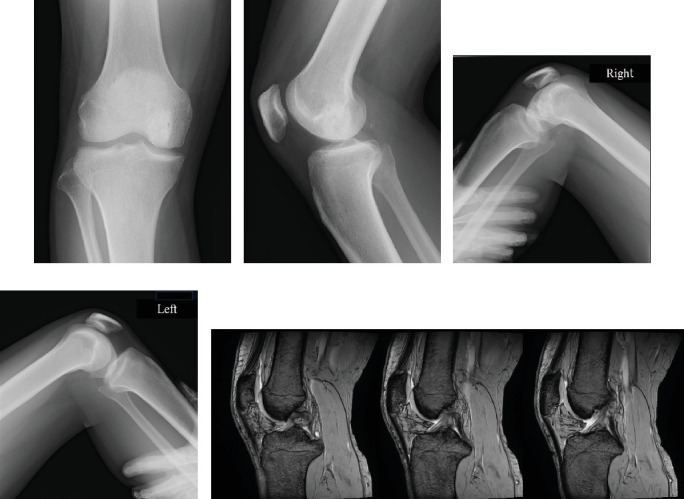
Frontal (a) and lateral (b) views of radiographs showing no signs of arthritic change or collapse of the femoral articular surface at 16 months' follow-up. PCL stress radiographs did not show any signs of increased posterior tibial translation of the affected right knee (c) compared to the left knee (d). Sagittal view of the magnetic resonance image (T2-weighted image) confirmed that the continuity of the PCL was well preserved (e).
